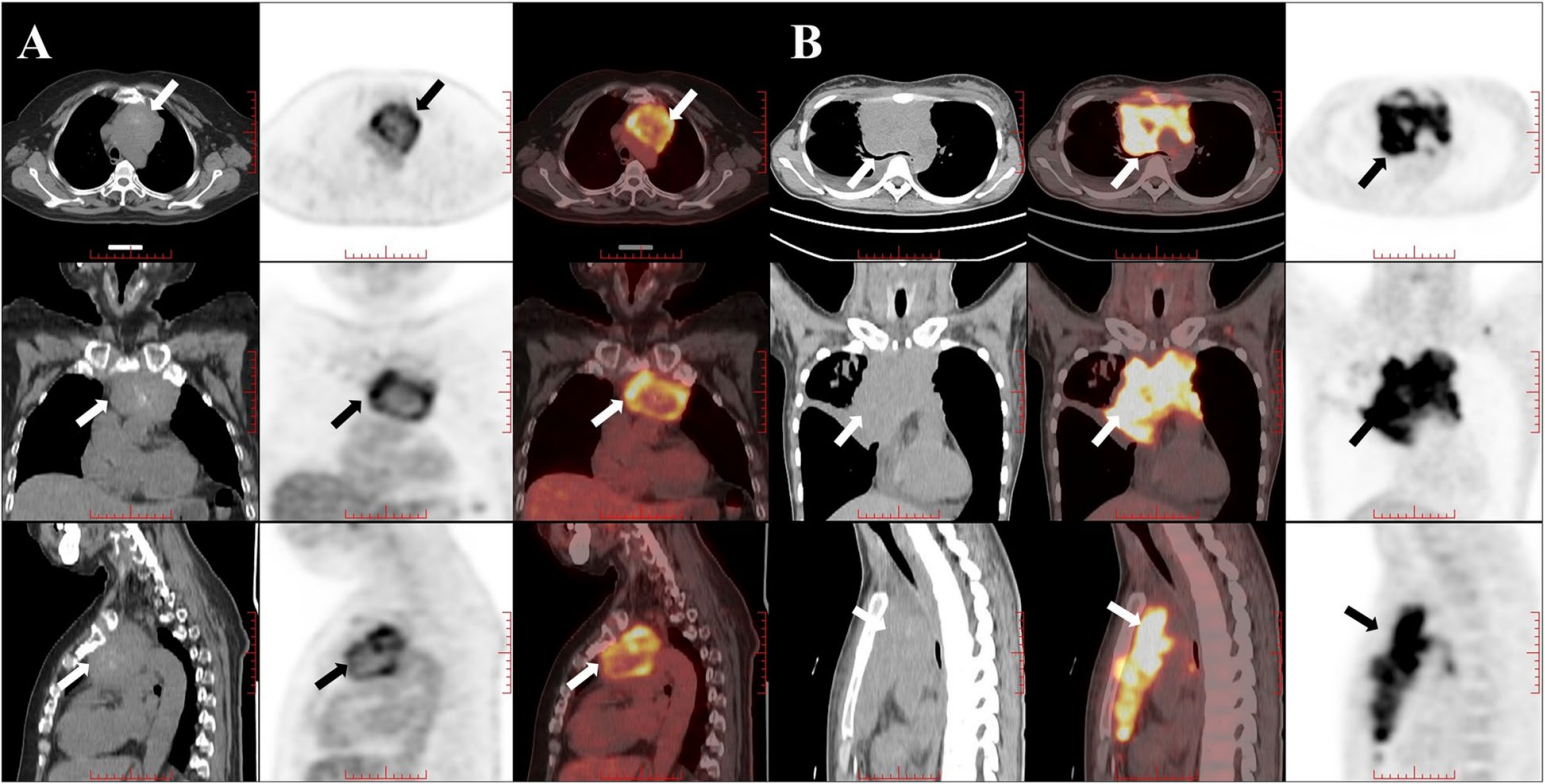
Image A in 59-year-old man with thymic squamous cell carcinoma (Masaoka Stage IIIB) in the anterior mediastinum (arrow). Enhanced CT showed that the boundary between the lesion and the blood vessel was not clear. Patient had chest and back pain. The lesion showed that SUVR was 2.49. Image B in 24-year-old woman with mediastinal diffuse large B-cell lymphoma (Ann Arbor Stage IVB) in the anterior mediastinum (arrow). Patient had respiratory symptoms (chest stuffiness) and B symptoms. The lesion showed that SUVR was 10.99
